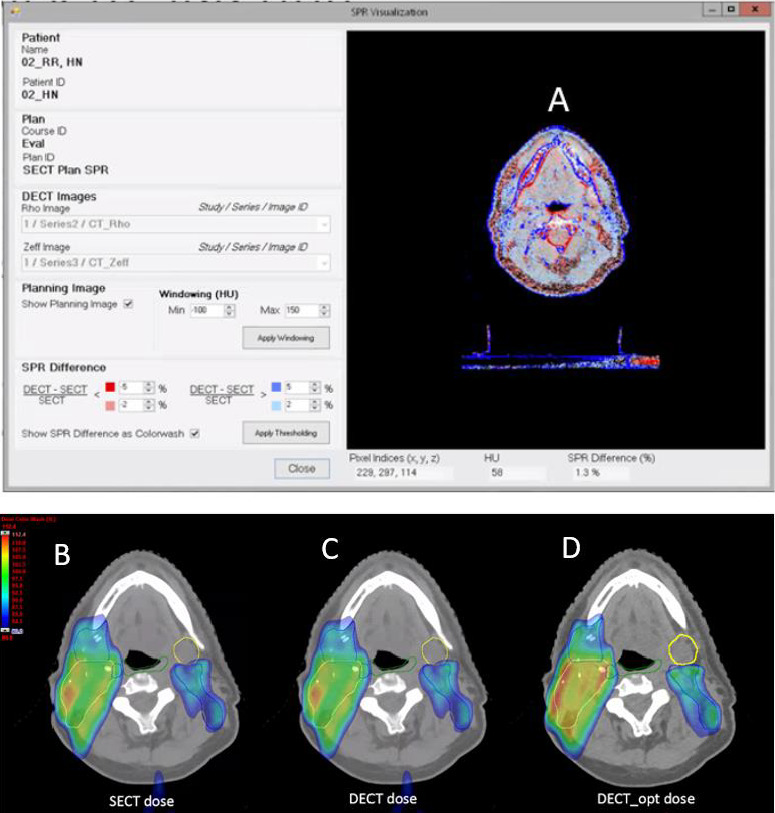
(A) Color map showing stopping power ratio (SPR) differences between dual- and single-energy computed tomography (DECT and SECT) for head and neck treatment. Blue (red) region shows higher (lower) DECT SPR compared with SECT. (B–D) Axial view of head and neck SECT dose (B), the corresponding forward calculated DECT dose (C), and the DECT-optimized dose (D). Yellow and blue target contours are clinical target volume (CTV) 6300 and CTV 5400, respectively. Organ-at-risk (OAR) contours for constrictors (green) and left submandibular (yellow) are shown.
